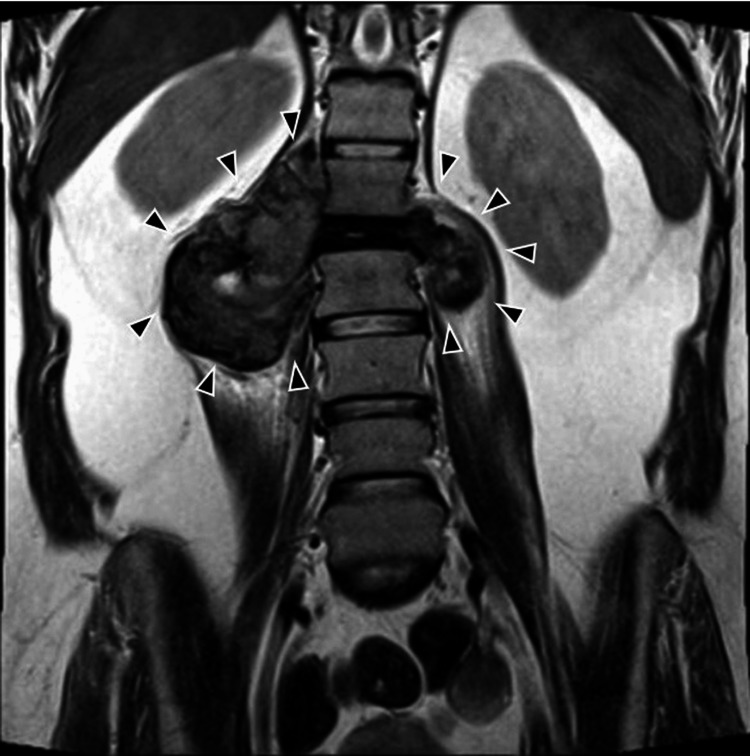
Preoperative coronal MRI of the lumbar spine, demonstrating a space-occupying lesion MRI, coronal view, T2-weighted sequence showing bilateral invasive expansion at the T1 vertebral body level, with a right-dominant mass effect and invasion of the iliopsoas muscle. The arrowheads delineate the lesion.
